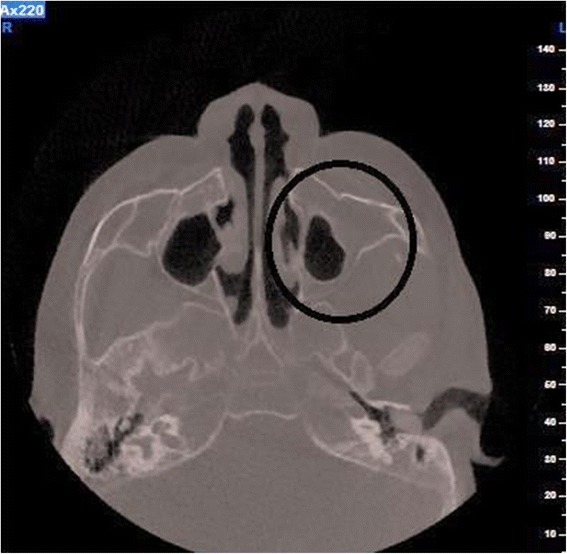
Cone Beam Tomography showing hypoplasia of both maxillary sinuses
